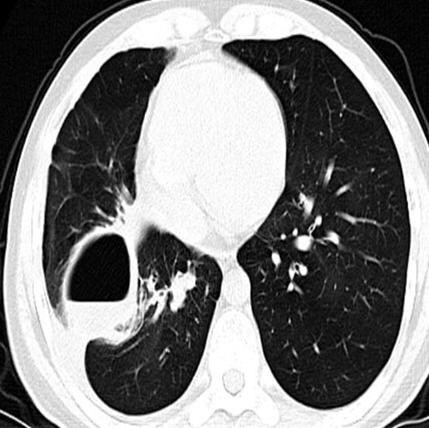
Chest CT shows the right lower lobe re-expansion, the bleb in right middle lobe aggravated into a large cyst with thick wall and fluid-level.
